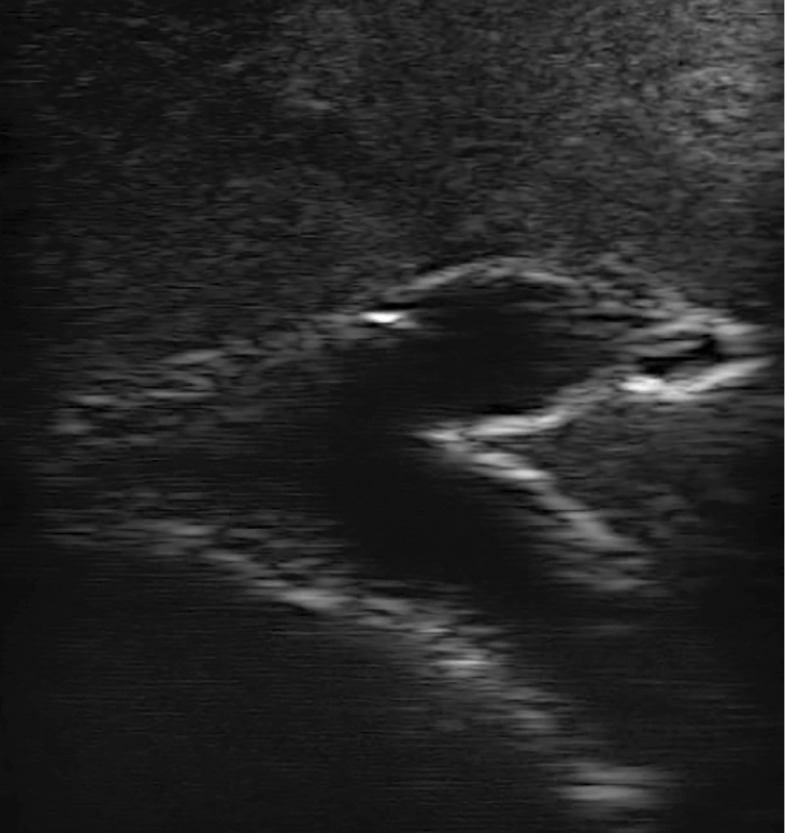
Ultrasound showing a vessel bifurcation with infiltrating hepatocellular carcinoma tissue
